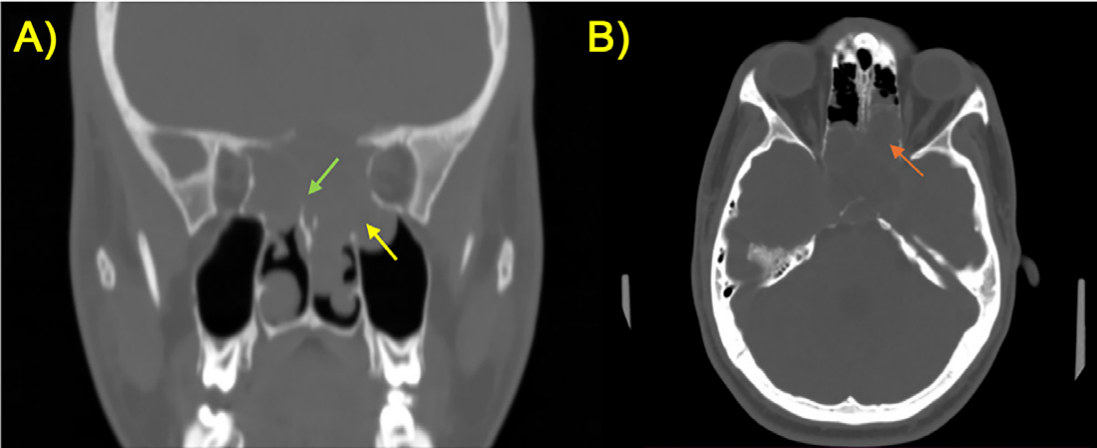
(A, B) CT scan on coronal (A) and axial (B) planes with bone window showed the erosion of the narrowing structures, in particular involving the sellar floor, posterior ethmoid (orange arrow), nasal cavity (green arrow), and left maxillary sinus (yellow arrow).
